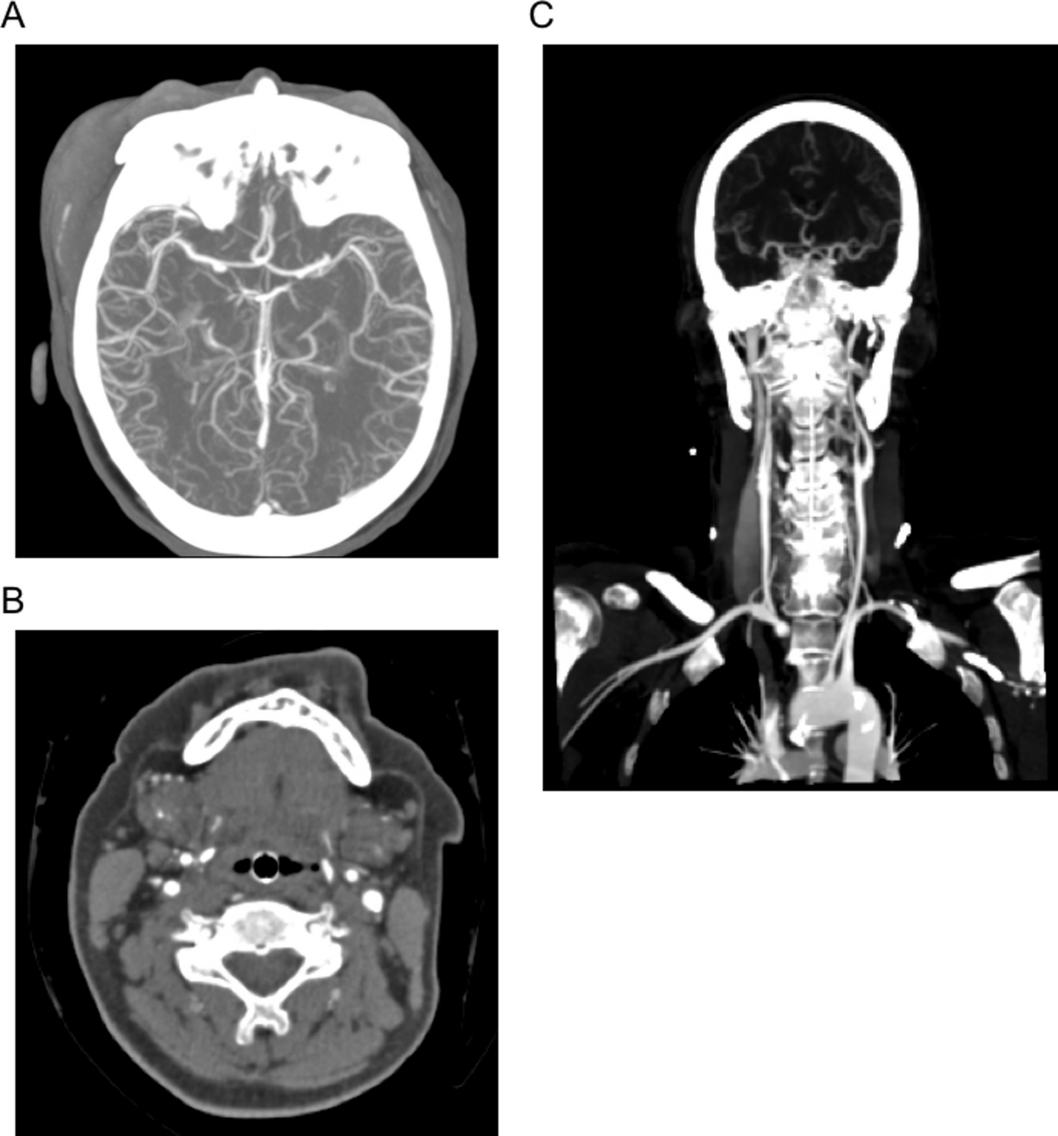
CTA of the head and neck via i.o. access. (A) Maximum intensity projection (MIP) axial reformatted image of the cerebral arteries with excellent enhancement and delineation up to distal segment levels. (B) Very good image quality with excellent enhancement of internal and external carotid arteries. (C) Maximum intensity projection (MIP) coronal reformatted image of the supraaortic branches.
